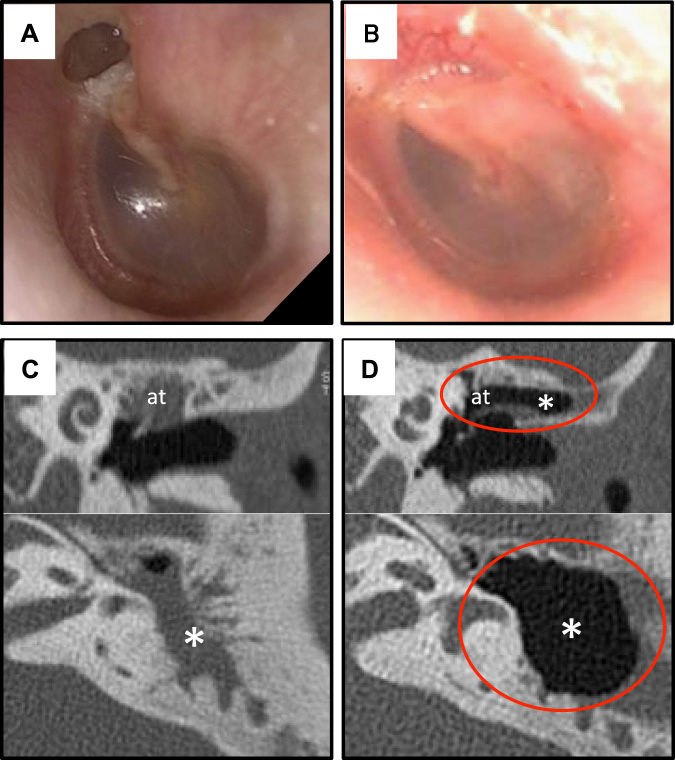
Photographs and CT images of the tympanic membrane and temporal bone before and after cell sheet transplantation (case 1). a Image of the tympanic membrane before surgery. There is retraction in the attic of the tympanic membrane. The image shows pars flaccida cholesteatoma. b An image of the tympanic membrane 12 months after surgery shows a favorable surgical outcome, without recurrence. c Preoperative CT images of the tympanic cavity and mastoid cavity: the upper image is the coronal view and the lower image is the axial view. Pneumatization is inhibited. There is no air (*black space*) visible in the attic (at) of the tympanic cavity or mastoid cavity (*). d CT images 12 months after surgery: the upper image is the coronal view and the lower image is the axial view. There is a well-pneumatized space in the region between the attic of the tympanic cavity and the mastoid cavity (indicated by *red* circles). CT: computed tomography
